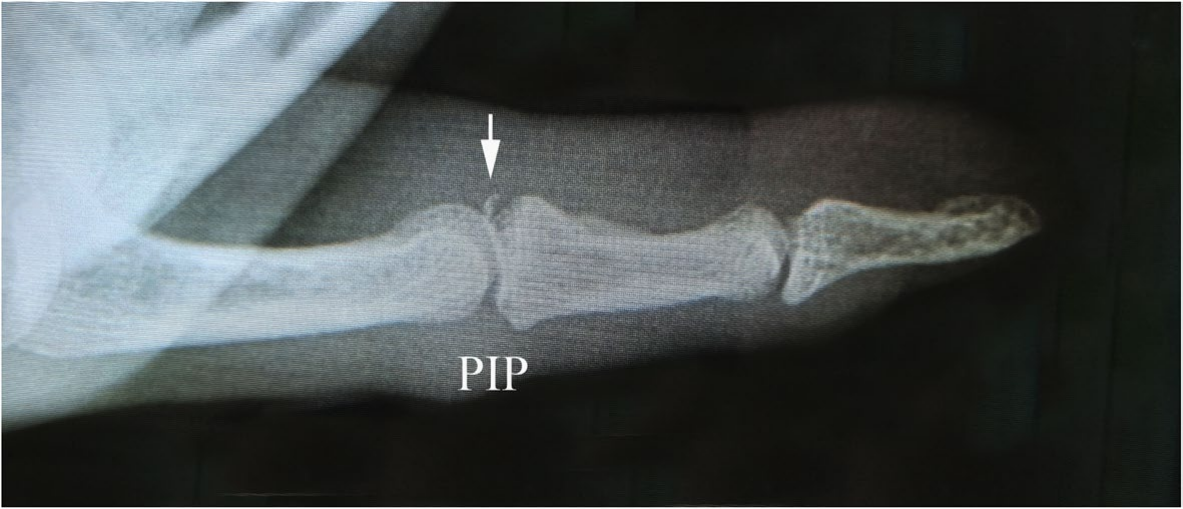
The X-ray findings of the same patient in Fig. 2 with type A volar plate injury. The arrow shows the avulsion bony fragment of the middle phalangeal base. PIP: proximal interphalangeal joint
